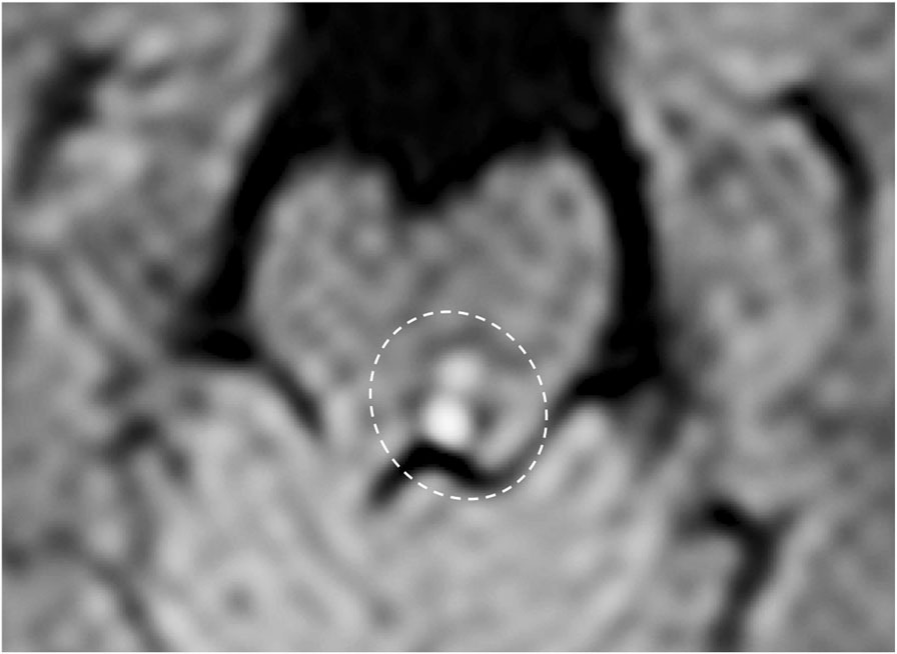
Trochlear nucleus ischemia. Patient with diplopia. MRI DWI (b1000) image shows restriction of the diffusion in the left posterior midbrain, in correspondence of the trochlear nucleus, suggestive for recent ischemic lesion (dotted circle)
